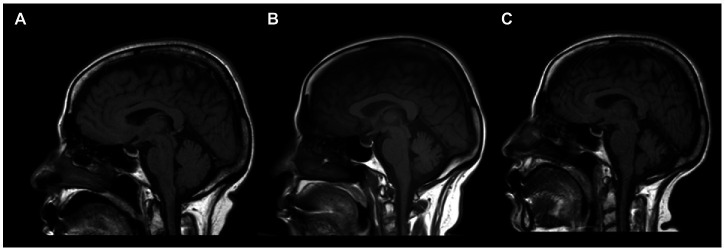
Sagittal brain MRI of an anti-mGluR1 encephalitis patient showing T1 sequence of (A) the cerebellar hemisphere upon initial presentation, which later showed progressive cerebellar atrophy at 10 months (B) and 20 months (C) of follow-up.
